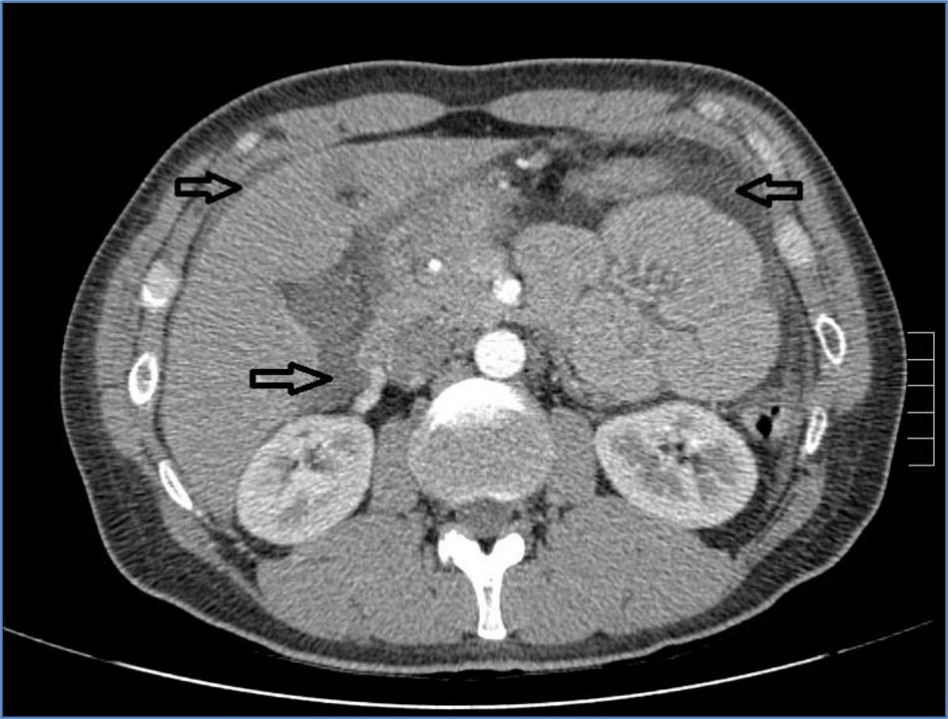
Abdominal CT scan showing diffuse fluid collection (arrows).
